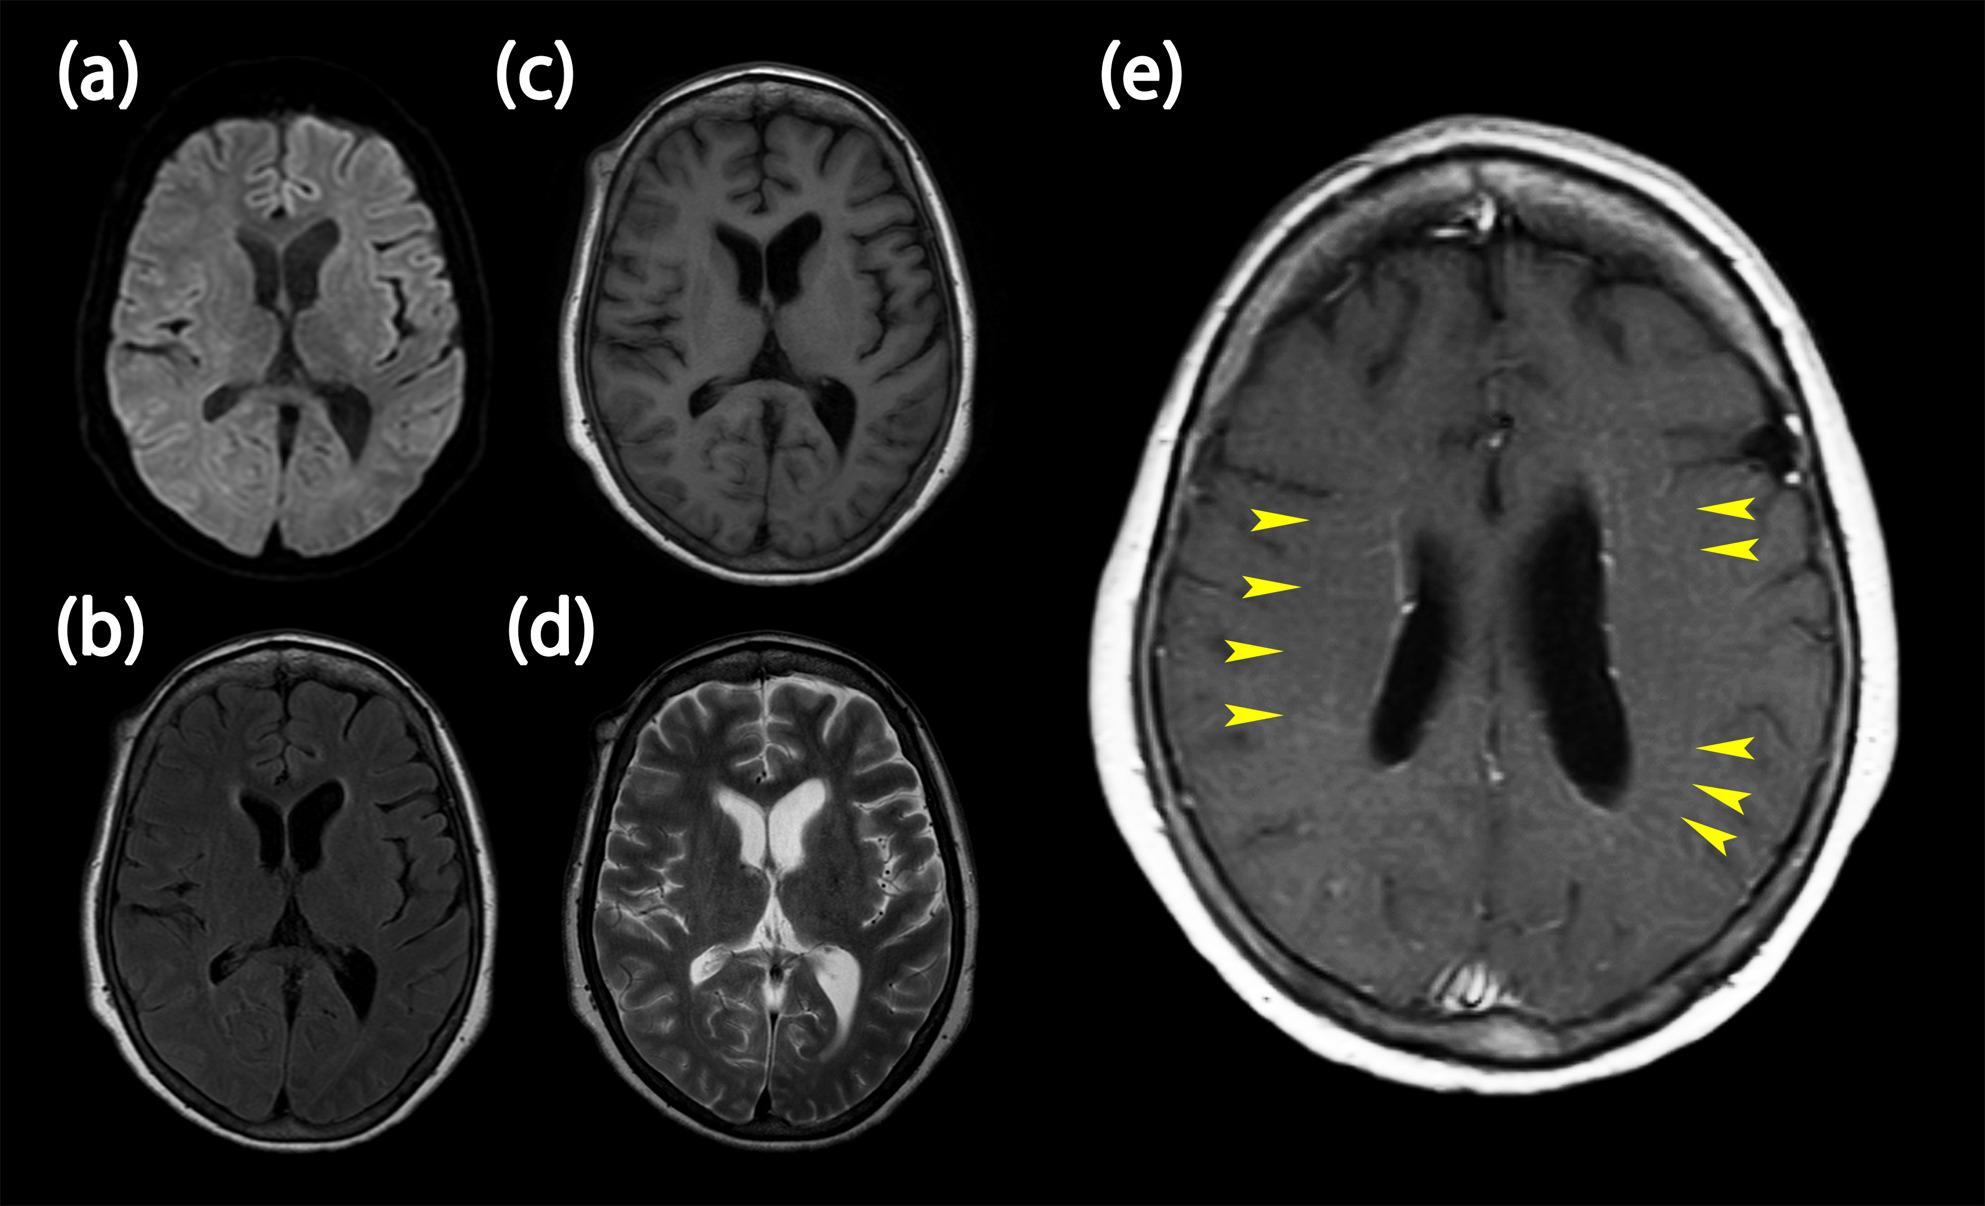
Brain MRI showing linear perivascular enhancement around the lateral ventricles. Brain MRI obtained on day 30: (a) diffusion-weighted imaging (DWI), (b) fluid-attenuated inversion recovery (FLAIR), (c) T1-weighted, (d) T2-weighted, and (e) contrast-enhanced T1-weighted sequences. Yellow arrowheads indicate the characteristic linear perivascular (radial) enhancement extending from the lateral ventricles, which is typical of GFAP astrocytopathy
